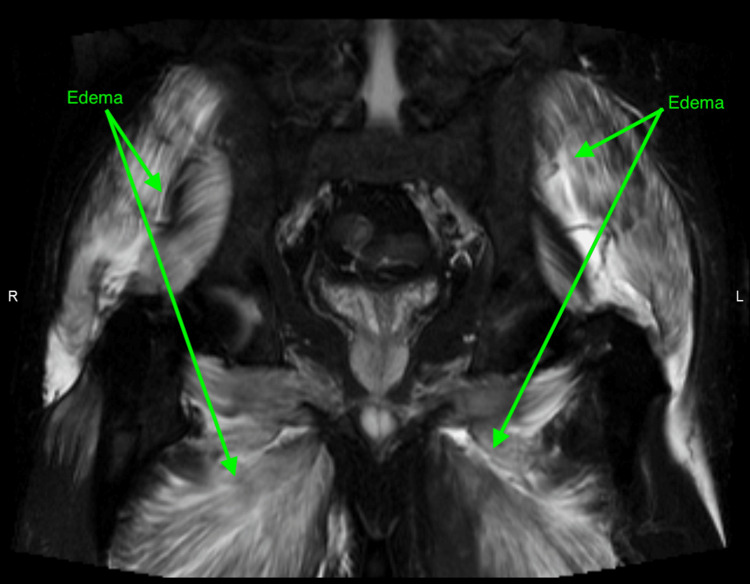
MRI of the pelvis without contrast - coronal STIR MRI: magnetic resonance imaging; STIR: short-tau inversion recovery
